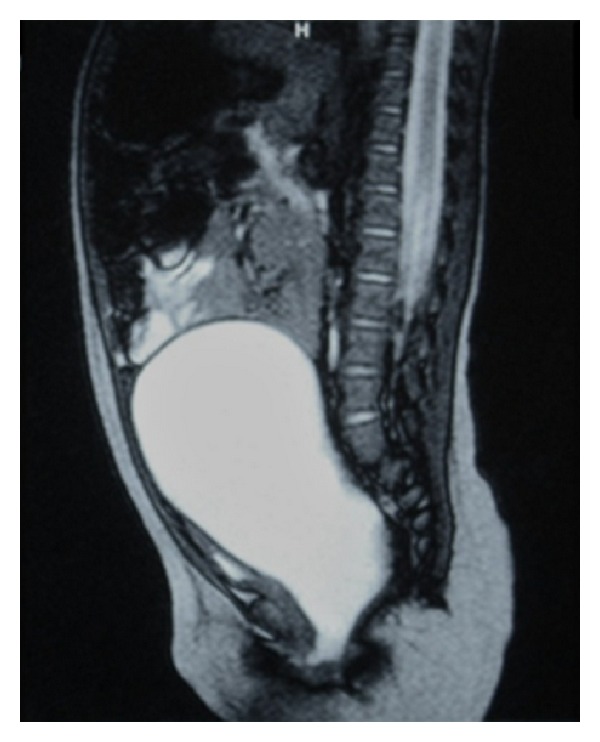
Sagittal-T2-weighted MR image. Marked distention of the uterus and cervix is demonstrated. Note the compressed urinary bladder with little urine in it.
